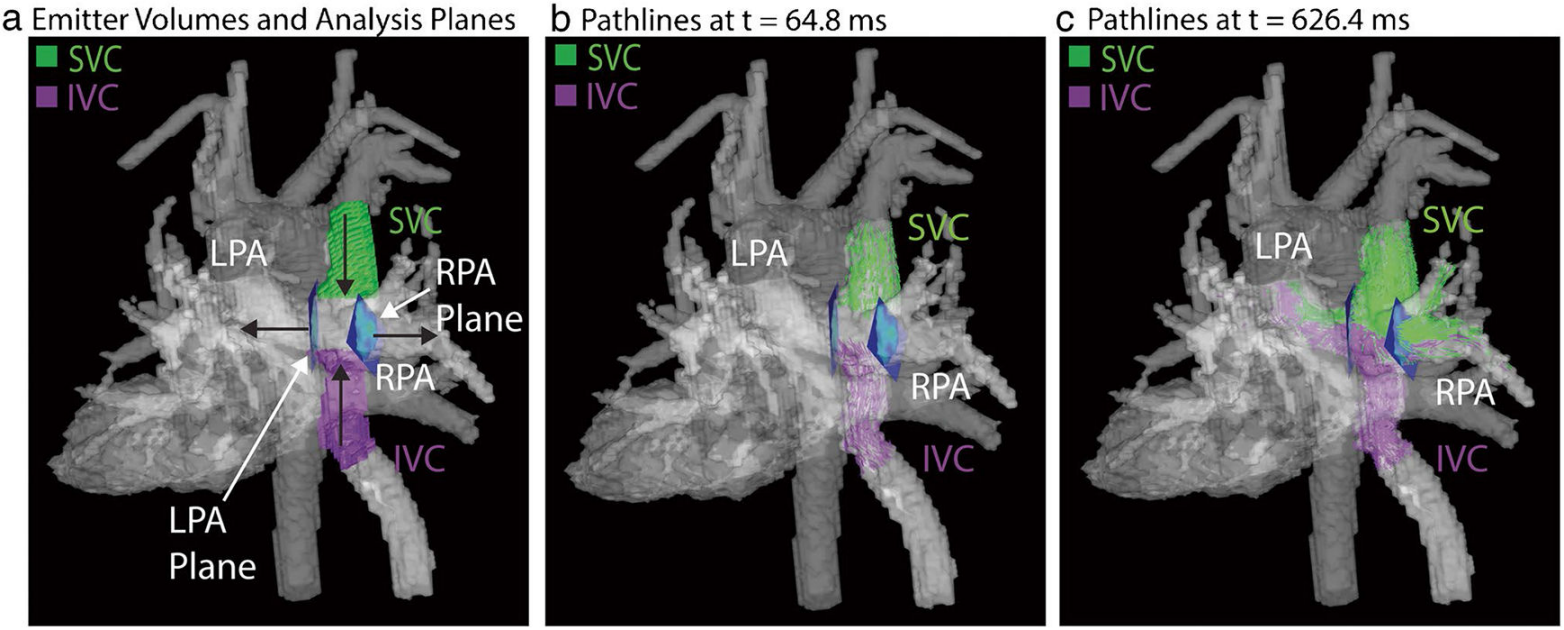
Flow distribution measurement. The IVC and SVC emitter volumes (purple and green, respectively) as well as manually placed analysis planes are shown in (a). Black arrows indicate the direction of flow within the total cavopulmonary connection. Pathlines at two progressive time points are shown in (b)–(c). Patient shown was 16 year-old male, status post extracardiac Fontan. Native anatomy included double outlet right ventricle and pulmonary valve atresia. Images depict segmentations derived from a phase contrast angiogram calculated from 4D flow MRI
